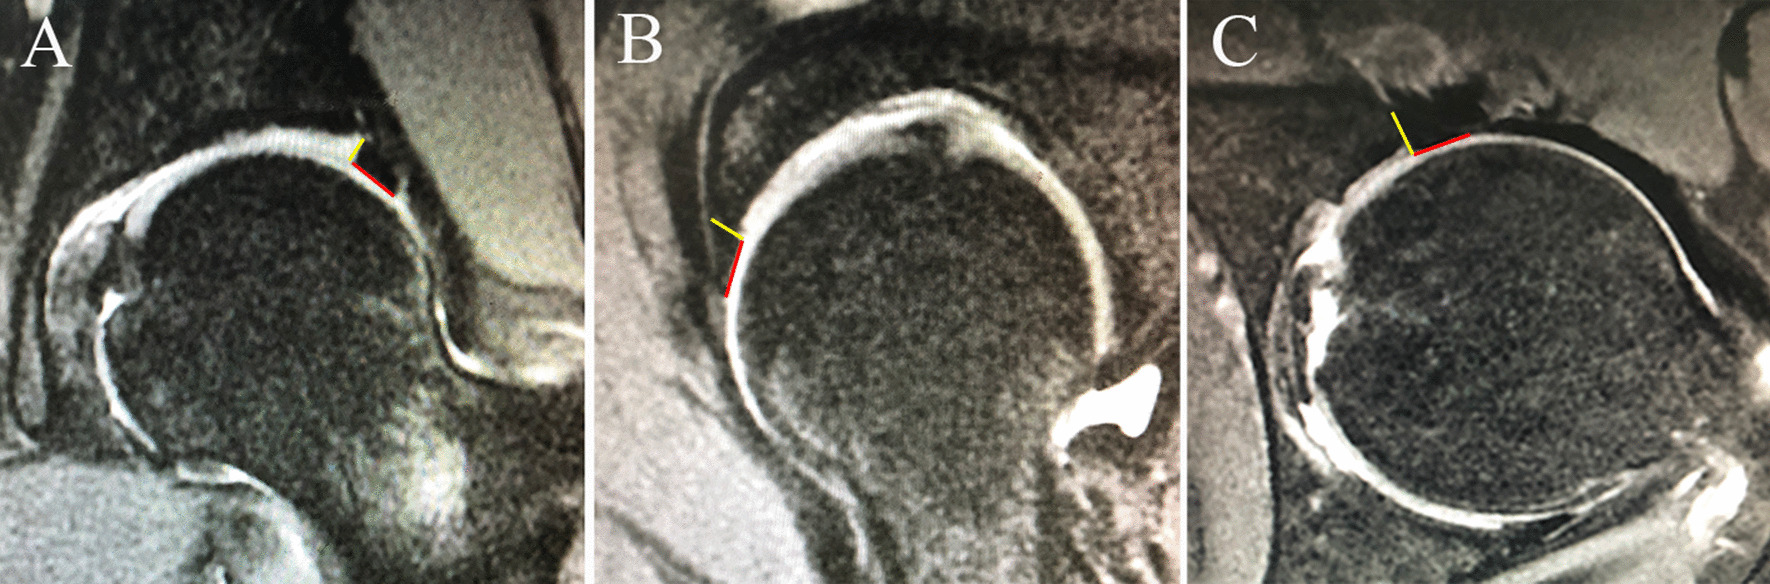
The labral length was measured from the chondrolabral junction to the tip of the labrum and labral height was the distance of the attachment measured from the joint surface to the perilabral recess utilizing a fat-saturated proton density (FSPD) sequence. The red line presents the length of the labrum and the yellow line presents the height of labrum. A Lateral labral length and height measurement technique on coronal magnetic resonance imaging (MRI). B Anterior labral length and height measurement technique on oblique sagittal MRI. C Anteroinferior labral length and height measurement technique on axial MRI
